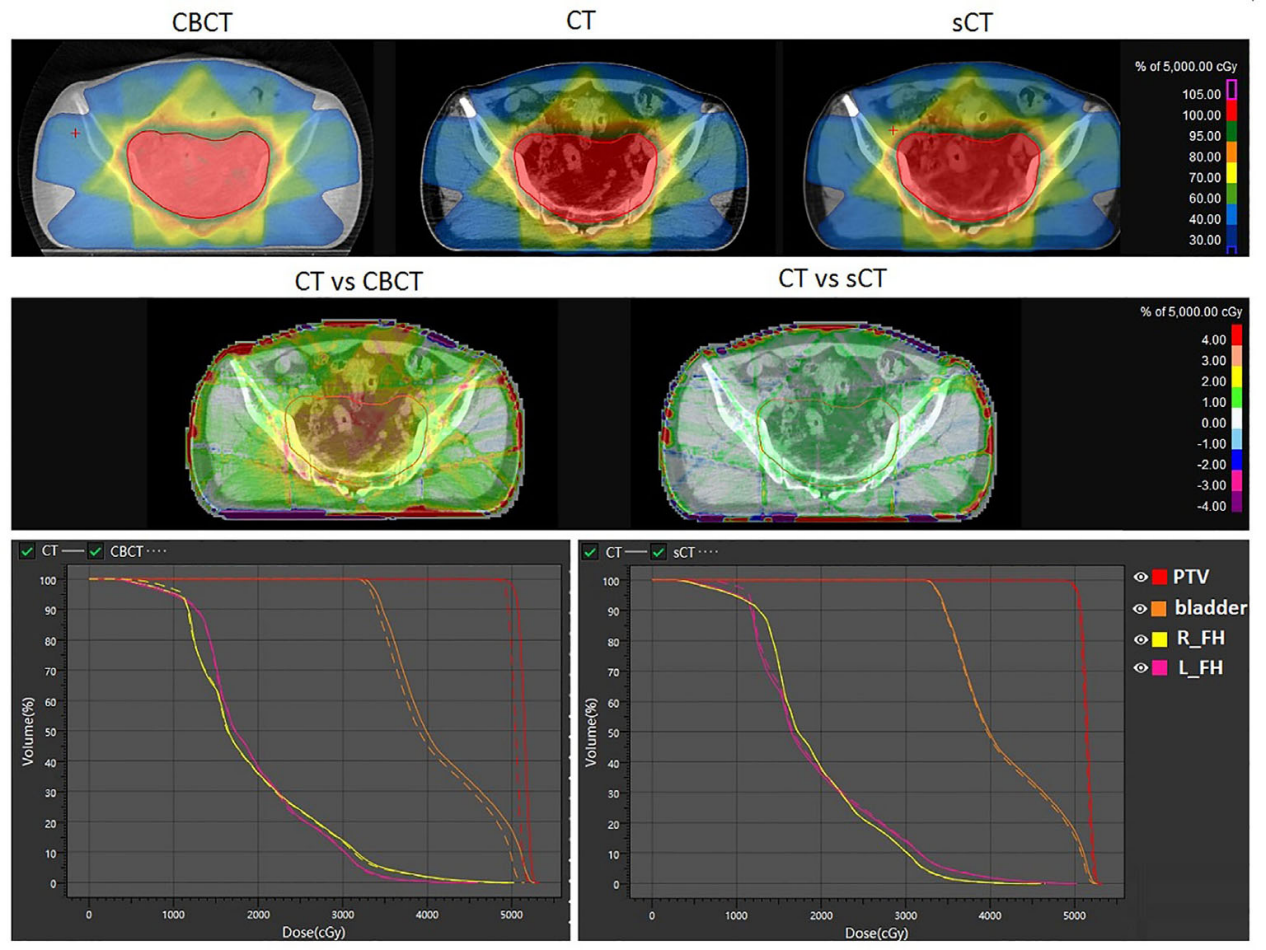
Dosimetric comparison of the same plan calculated on CBCT, sCT and CT. The first row shows the dose distribution on CBCT, CT and sCT. The second row shows the dose distribution differences. The third row shows the DVH differences. L_FH and R_FH means left femoral head and right femoral head.
